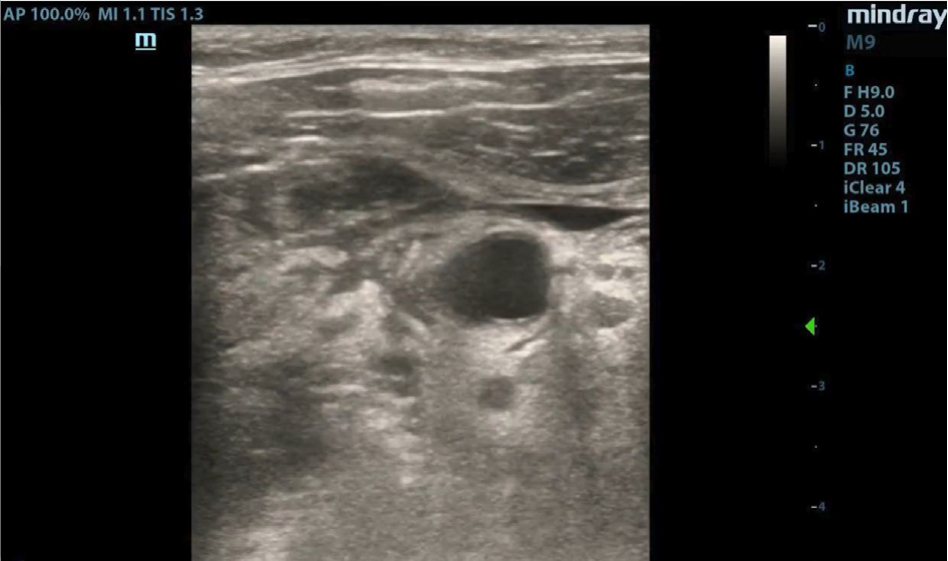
Ultrasound revealing subcutaneous air adjacent to the trachea.
